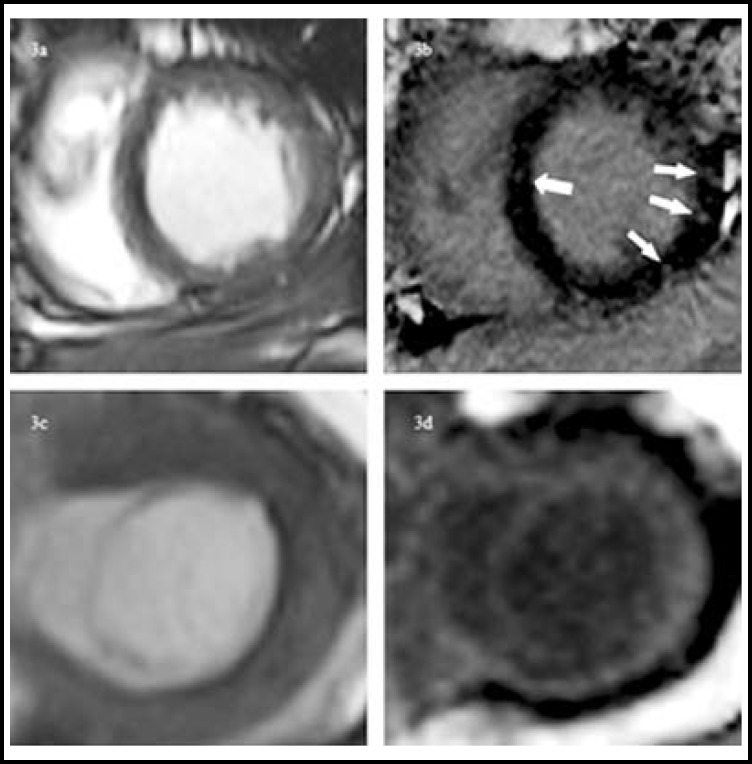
MR findings in 2 patients with systemic amyloidosis. Cine MRI (a, c) and LGE-MRI (b, d) in the cardiac short-axis plane. Image of 46-year-old man showed (b) focal delay enhancement (white arrows) and. Image of 57-year-old man showed (d) diffuse delay enhancement
